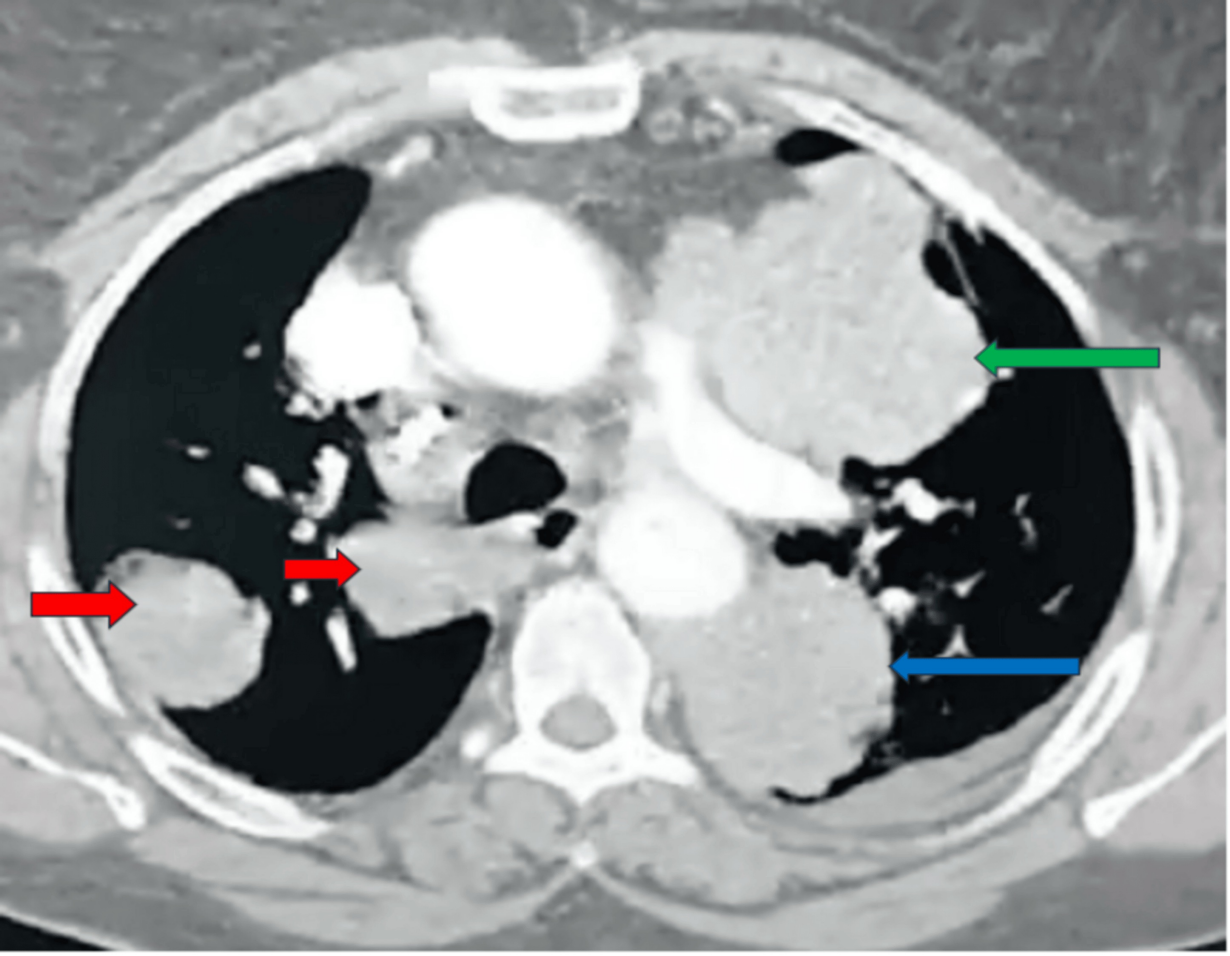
Thorax CT scan with mediastinal window demonstrating two voluminous lesion processes, one posteroapical on the left and the other anteroapical on the left, with the presence of multiple nodular and macronodular formations on the right, appearing secondary in nature. Blue arrow: Indicates a voluminous lesion process in the left posteroapical position, measuring 74 x 69 mm, with pleural involvement in its external part. Green arrow: Highlights a second, similar lesion process, located in the left anteroapical position, measuring 50 x 40 mm, grazing the hemi-circumference of the pulmonary artery trunk and presenting an external parenchymal component. Red arrows: Point to multiple nodular and macronodular formations on the right, the largest measuring 38 x 37 mm.
